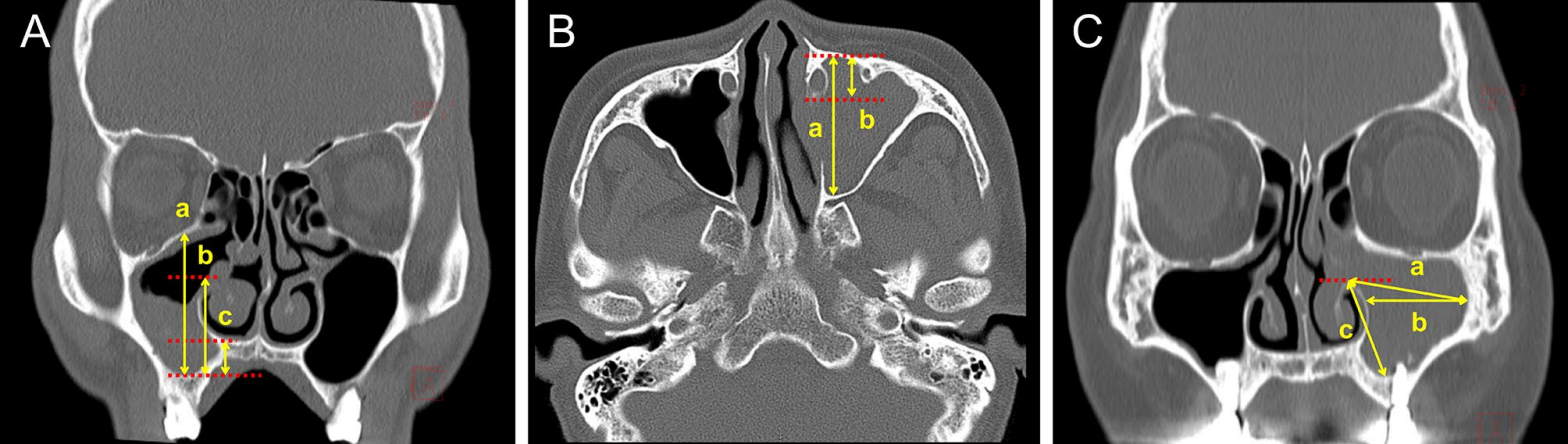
Parameters of maxillary dimensions. (A) Several parameters of maxillary dimensions were measured in PNS CT or OMU CT, estimated as (a) Maxillary sinus height (MSH), (b) Ostium height (OsH), and (c) Nasal floor height (NFH) in the coronal view. (B) (a) MS maximum anteroposterior distance (Max AP) and (b) Maximum anterior recess depth (Max ARD) were calculated in the axial view. (C) Estimation of (a) Maximum oblique width (Max OW), (b) Maximum horizontal width (Max HW), and (c) Distance from ostium to maxillary sinus inferior wall (OsI) was depicted in coronal view.
